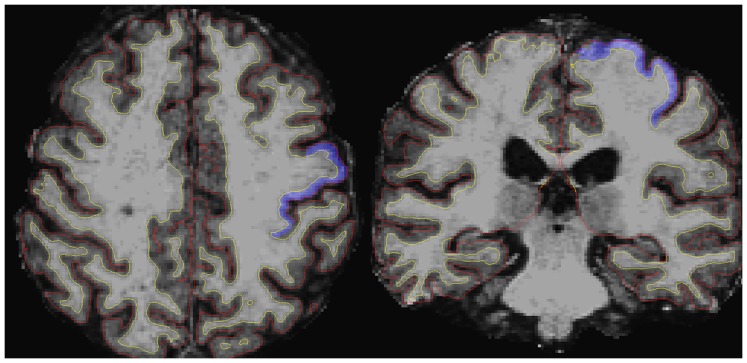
Example of the CTh boundaries of an ALS patients. Axial and coronal T1-weighted images of an ALS patient. White matter surface (yellow line) and pial surface (red line), which are the boundaries of cerebral cortex, have been automatically obtained by Freesurfer. The primary motor area (blu) has been automatically labeled according to the Desikan/Killiany Atlas.
